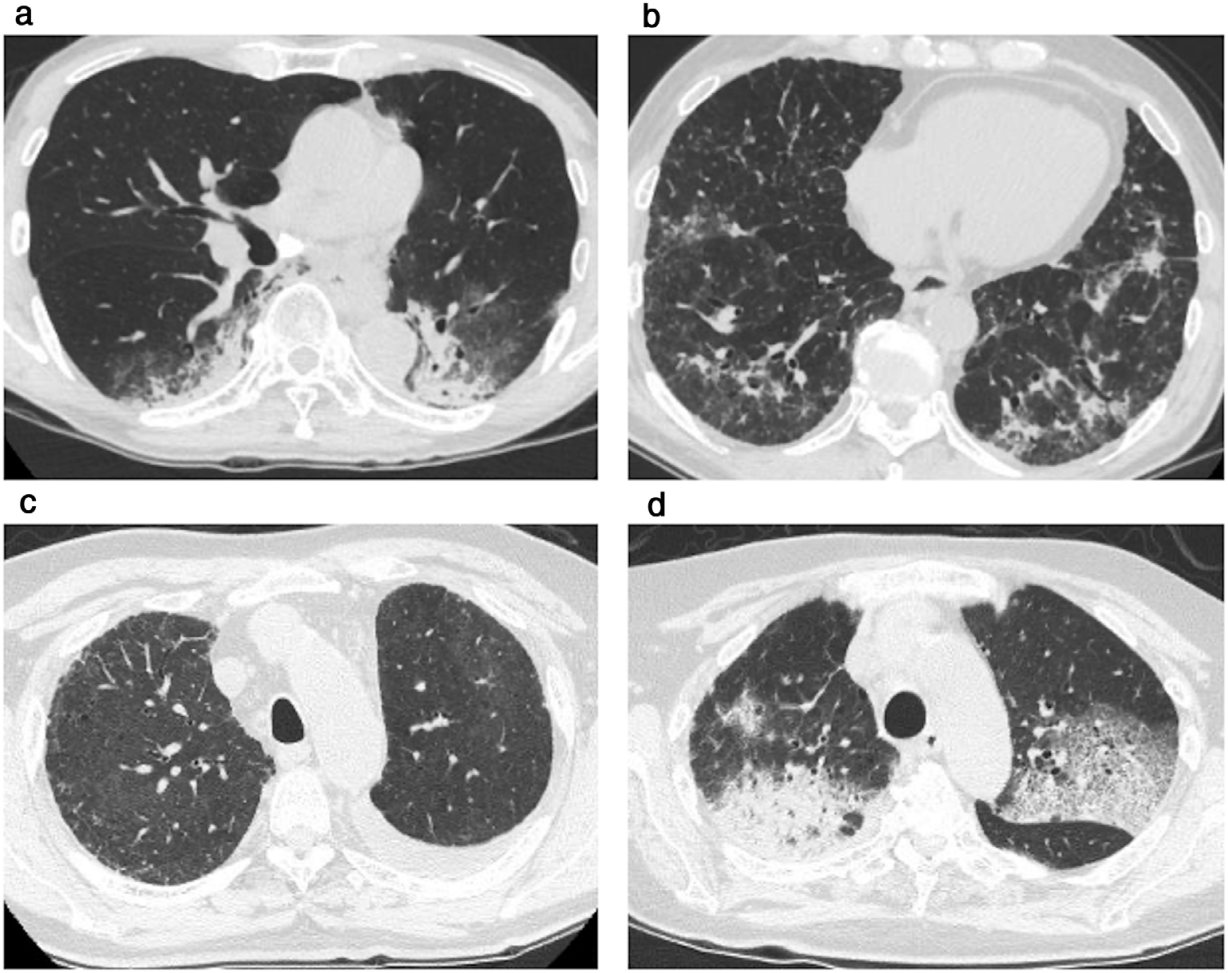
Radiographic pattern of immune checkpoint inhibitor (ICI)‐related pneumonitis (ICI pneumonitis. (a) COP pattern; (b) NSIP pattern; (c) HP pattern; and (d) AIP/ARDS pattern. COP, cryptogenic organizing pneumonia; NSIP, nonspecific interstitial pneumonia; HP, hypersensitivity pneumonitis; AIP/ARDS, acute interstitial pneumonia/acute respiratory distress syndrome.
